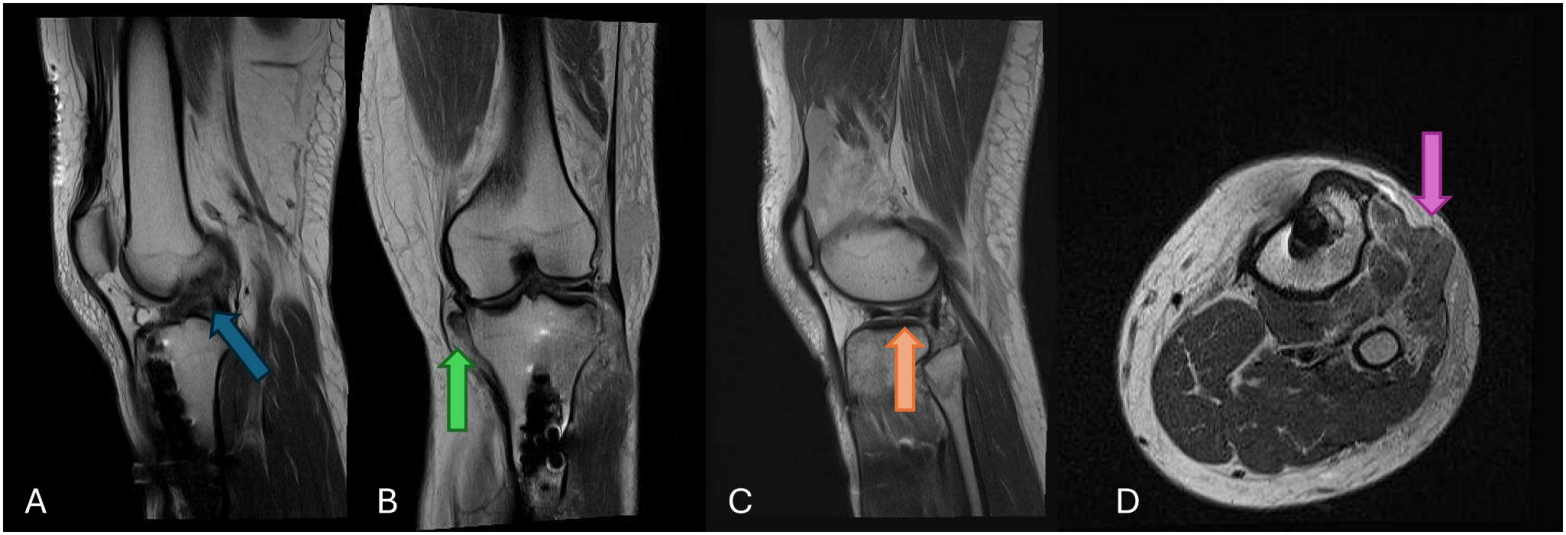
Patient presented in ER with poly trauma after motor vehicle accident. The baseline lower limb part of the whole body CT was limited due to artifacts. Due to high level of suspicion for soft tissue injuries MRI was performed which detected high grade partial thickness ACL tear (image A), complete tear of the distal MCL with retraction (image B), lateral meniscal tear (image C) and fascial tear of the anterior compartment of the proximal leg with herniation of the extensor digitorum longus and tibialis anterior muscles (image D).
